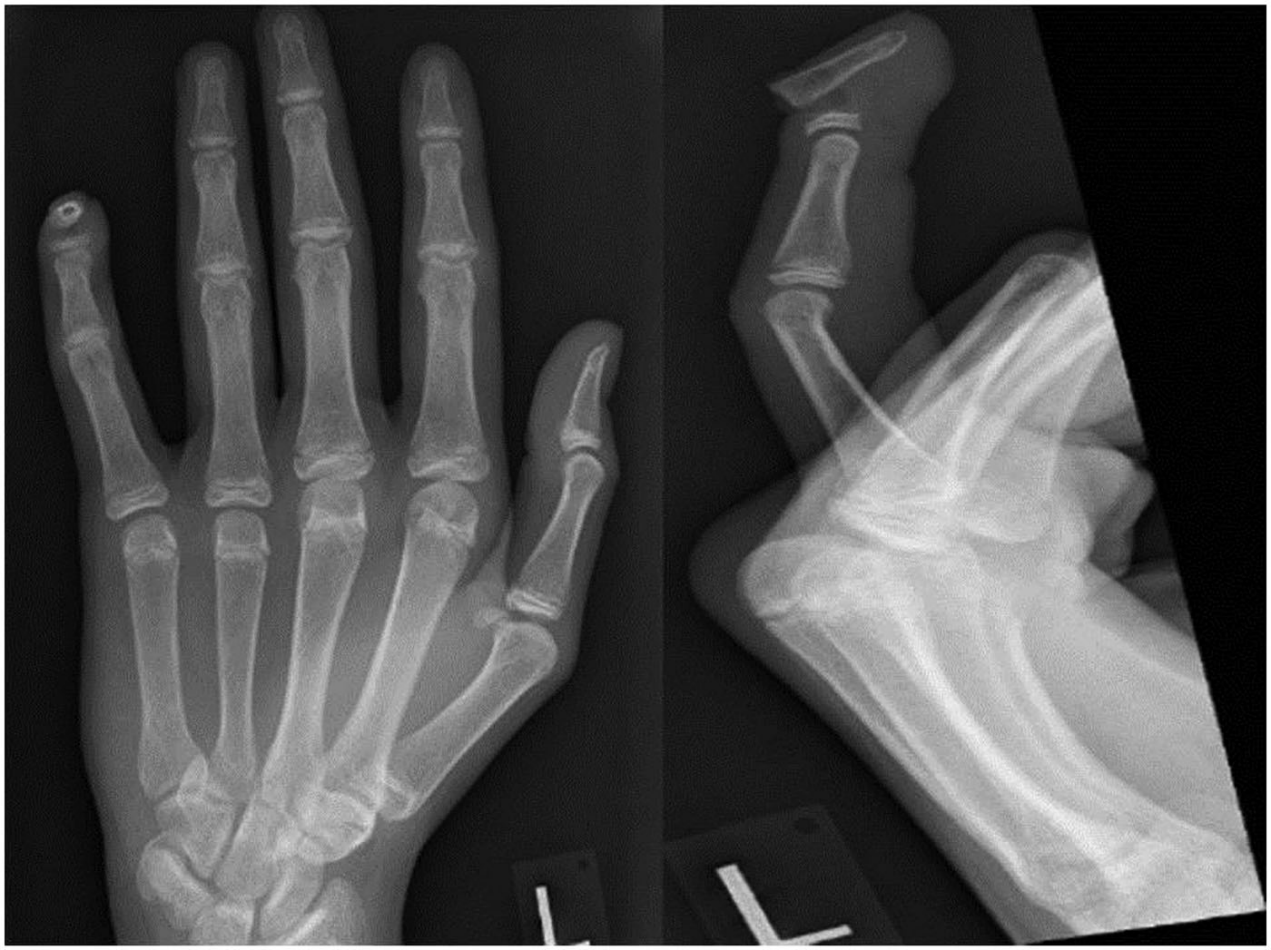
Left Little Finger AP + Lateral Radiographs – Seymour Fracture.
